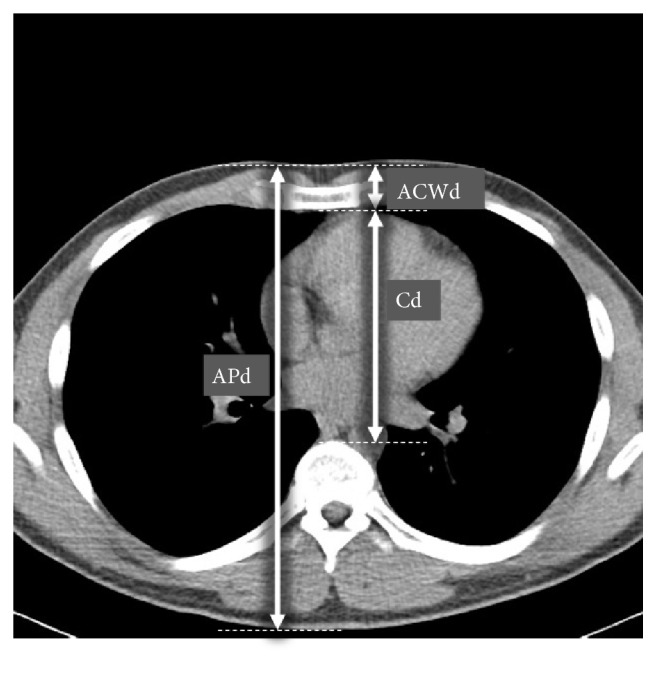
Measured diameters on an axial chest CT image at the level of the lower half of the sternum. APd was defined as the distance from the anterior skin to the posterior skin. ACWd was defined as the distance from the anterior skin to the posterior sternum. Cd was defined as the distance from the posterior sternum to the anterior vertebral body. APd: anteroposterior diameter; ACW: anterior chest wall diameter; C: compressible diameter.
